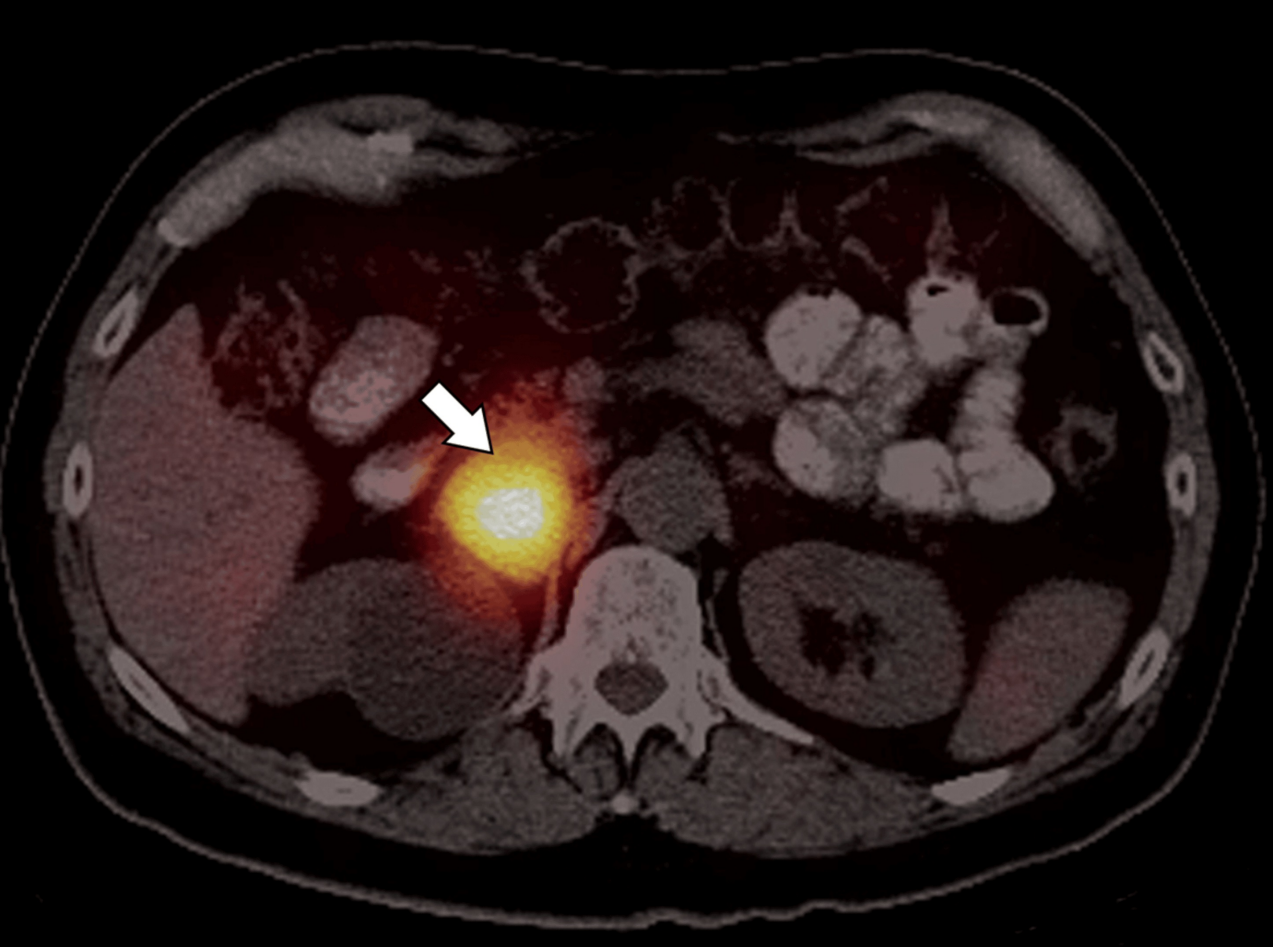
Axial ¹²³I-MIBG scintigraphy showing adrenal lesion uptake Axial iodine-123 metaiodobenzylguanidine (¹²³I-MIBG) single-photon emission computed tomography (SPECT) image demonstrates intense radiotracer uptake corresponding to the right adrenal lesion (arrow), consistent with a pheochromocytoma.
